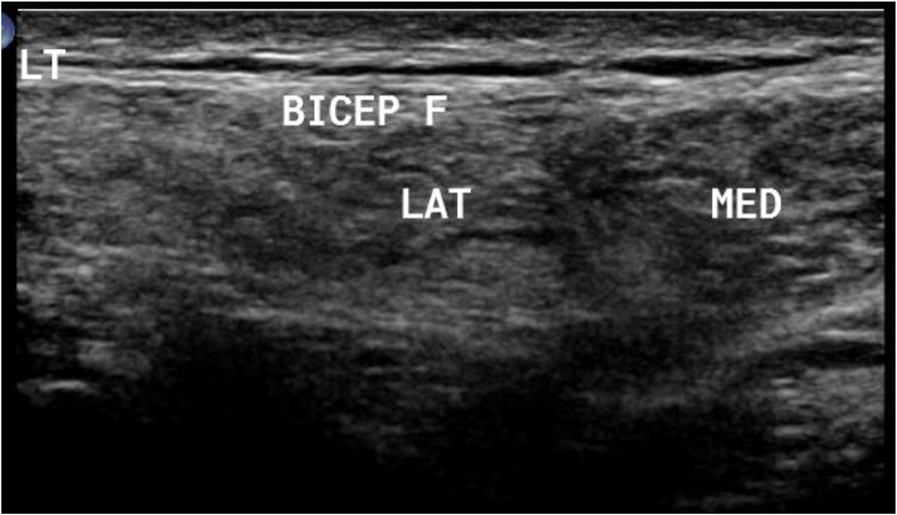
In patient with LGMD2B, ultrasound detected preferential affection of both heads of biceps brachii
